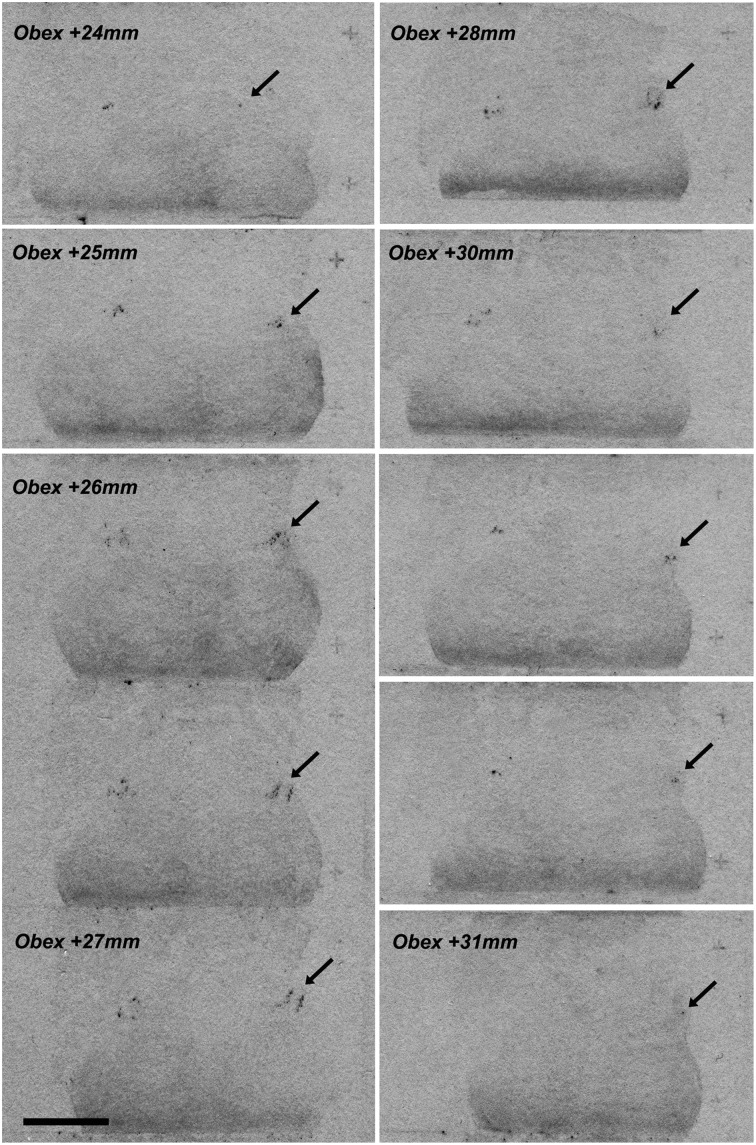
NPS mRNA-positive neurons at different rostro-caudal levels of human pons (X-ray film). A series of coronal brainstem sections, from Obex +24 to Obex +31, hybridized with ^35^S-labeled hNPS riboprobes and exposed to KODAK X-ray film. A very limited but distinct signal is detected bilaterally in the proximity of the superior cerebellar peduncule/parabrachial region (arrows). Two subclusters of labeled cells are seen around Obex +27 mm. Scale bar: 10 mm.
